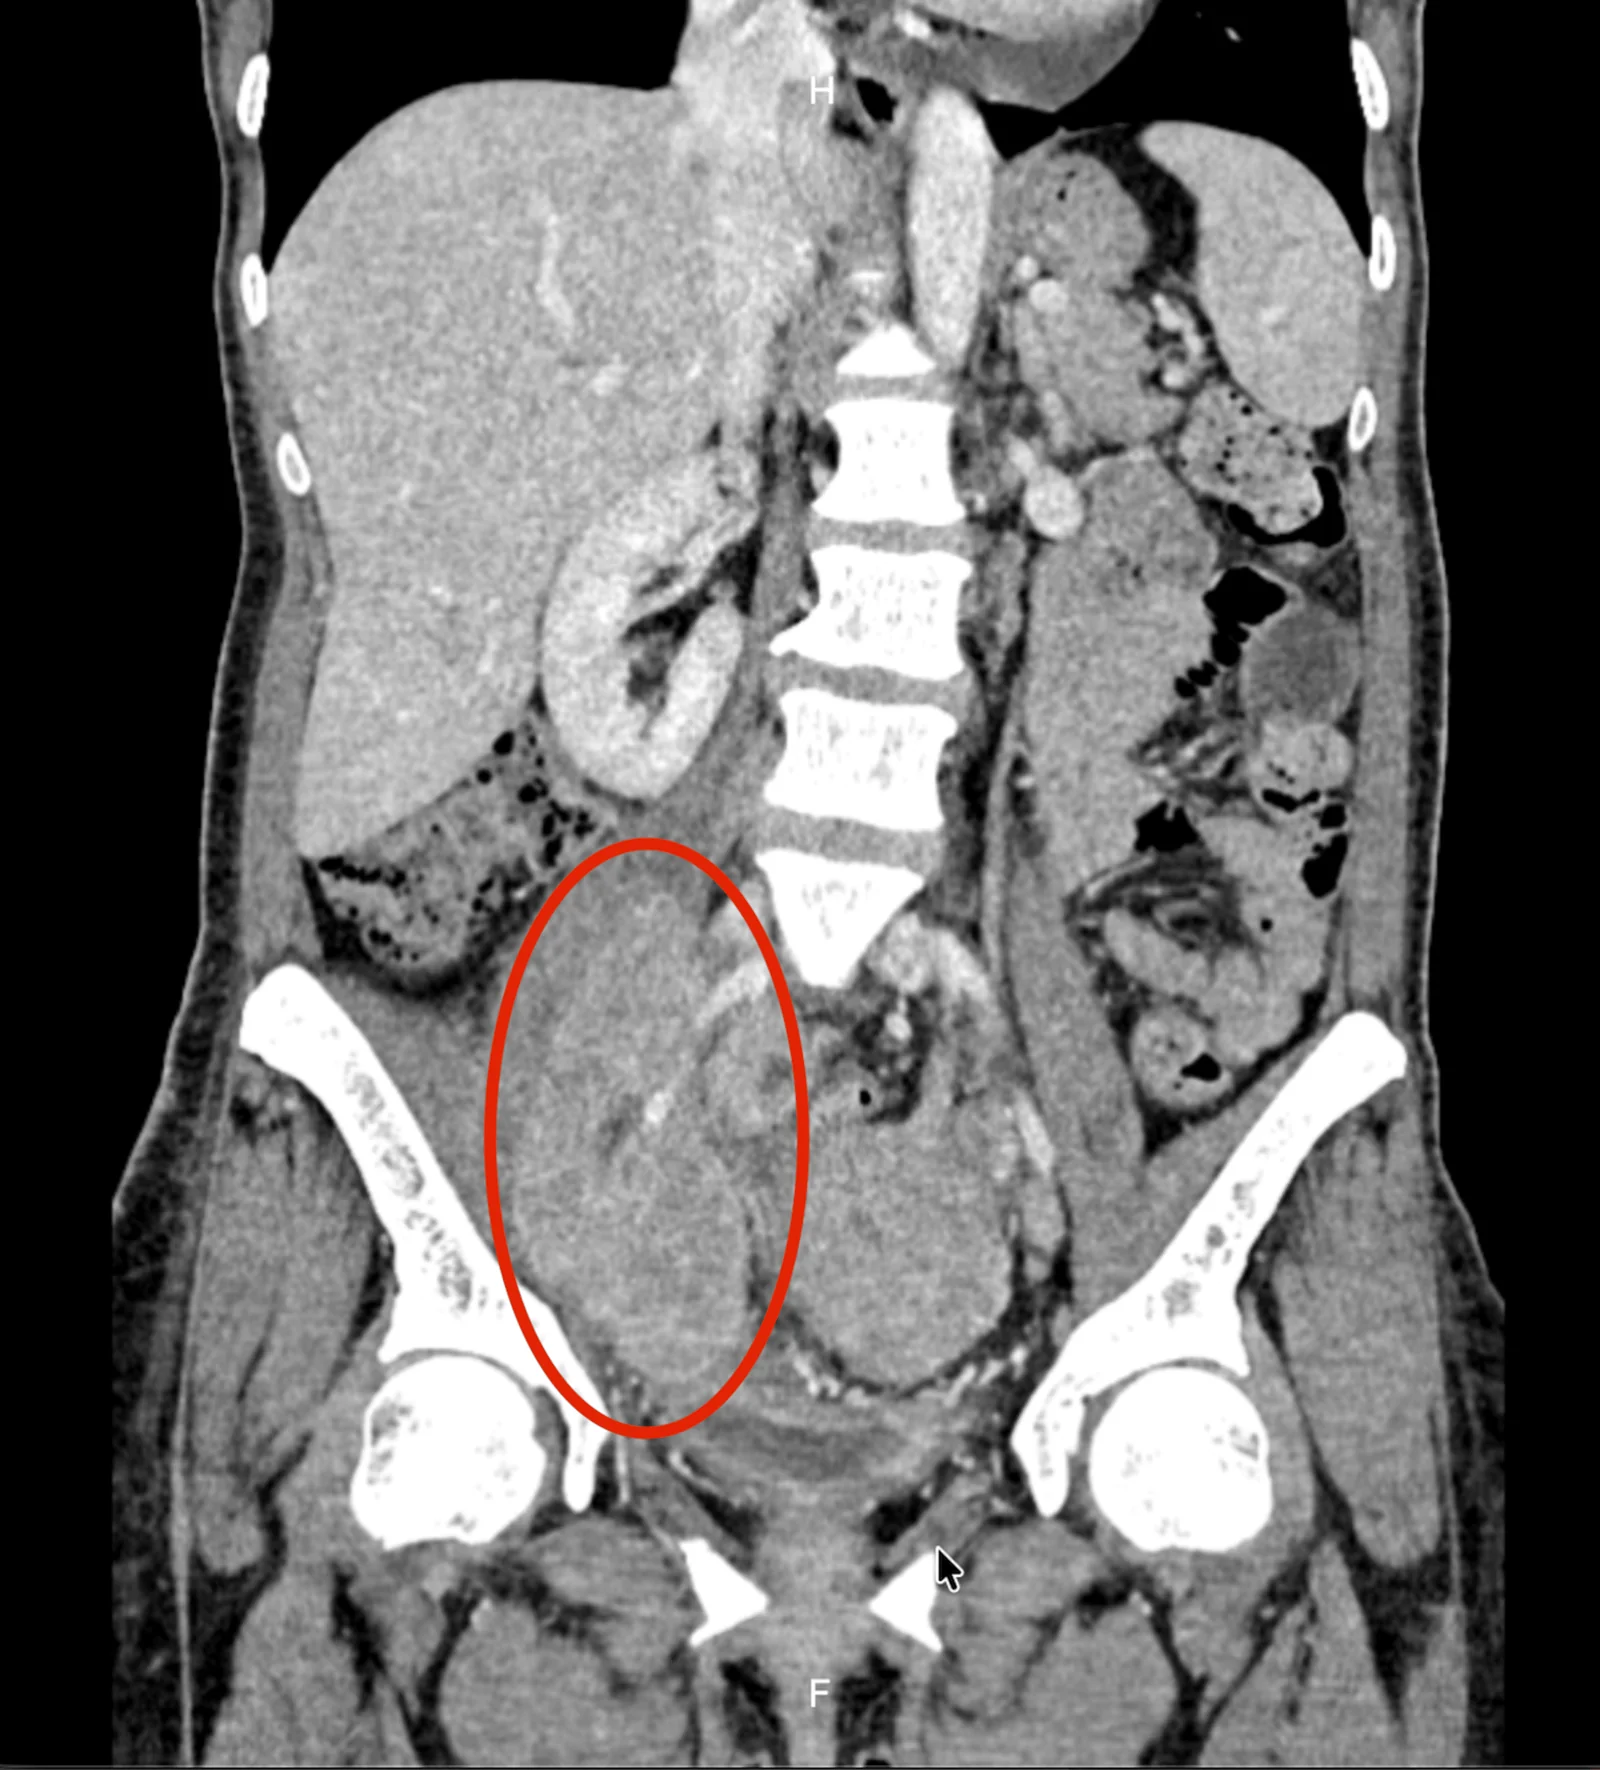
Abdominal and pelvic computed tomography in the coronal plane with intravenous contrast. Lesion suggestive of a collection extending from the right adnexal region toward the iliopsoas muscle, with heterogeneous post-contrast enhancement, indicated by an arrow. H: head; F: foot
